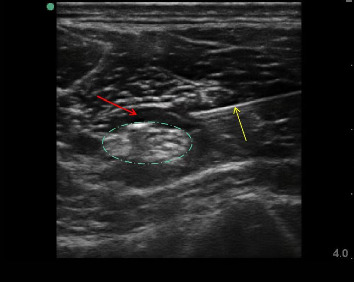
Ultrasound-guided imaging of platelet-rich plasma injections in the sciatic nerve. The high-echo image indicated by the yellow arrow shows the injection needle, the echo-less image indicated by the red arrow shows platelet-rich plasma, and the high-echo image within the blue oval line indicates the sciatic nerve.
